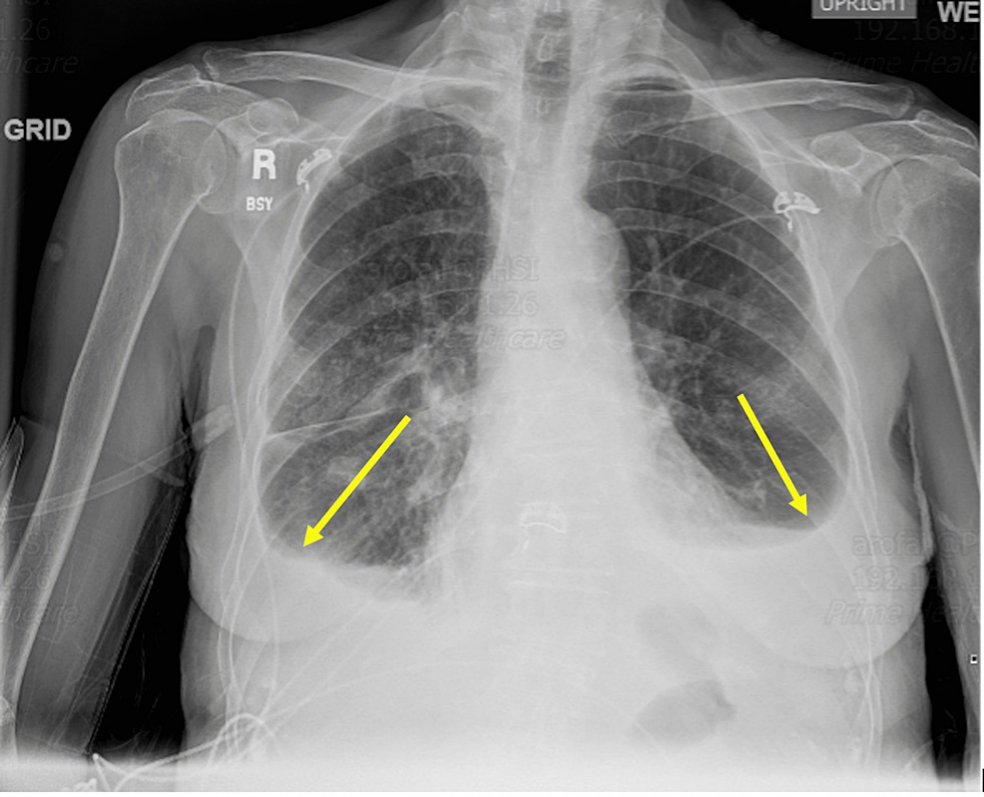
Chest X-ray showing reduction of left-sided pleural effusion after thoracentesis.
